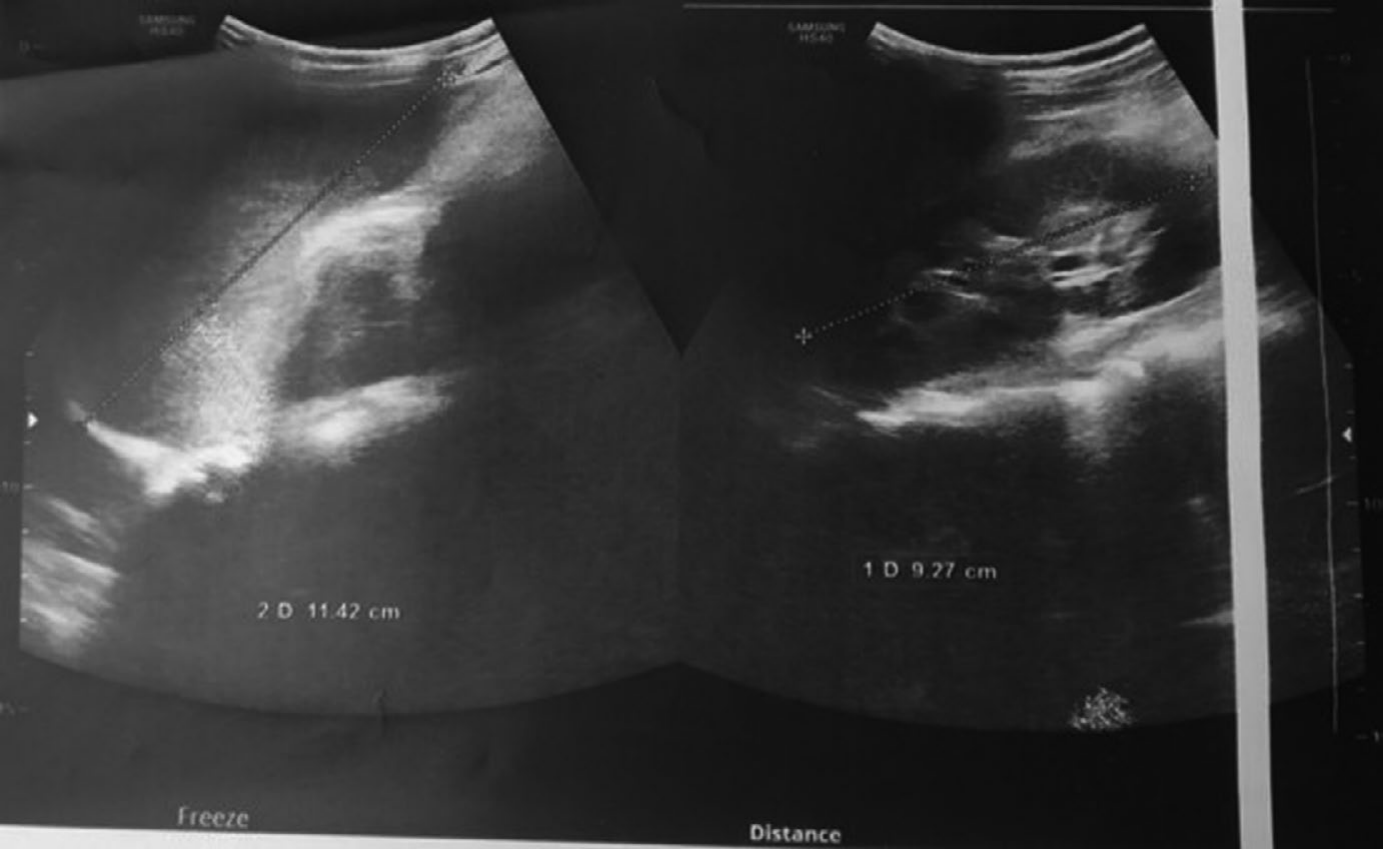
Ultrasonography showing hepatomegaly with coarse echotexture as compared to renal cortex
